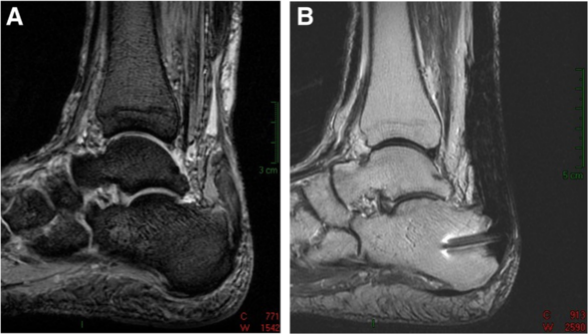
a and b MRI of a 72 year old patient, before surgery (9 months after Achilles tendon tear), and 8 months after surgery with autologous quadriceps tendon graft
